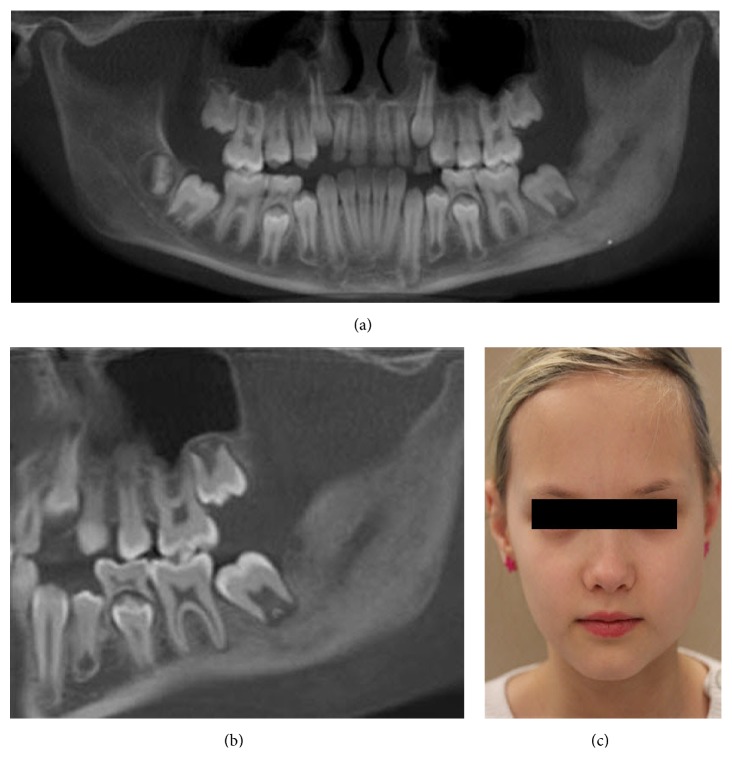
Six-month postoperative control CBCT and clinical image of patient case 1 with PCO. (a) Panoramic CBCT; (b) sagittal section of sclerotic mandible with no lytic lesion; and (c) patient photo, with reduced facial swelling at the left side.
